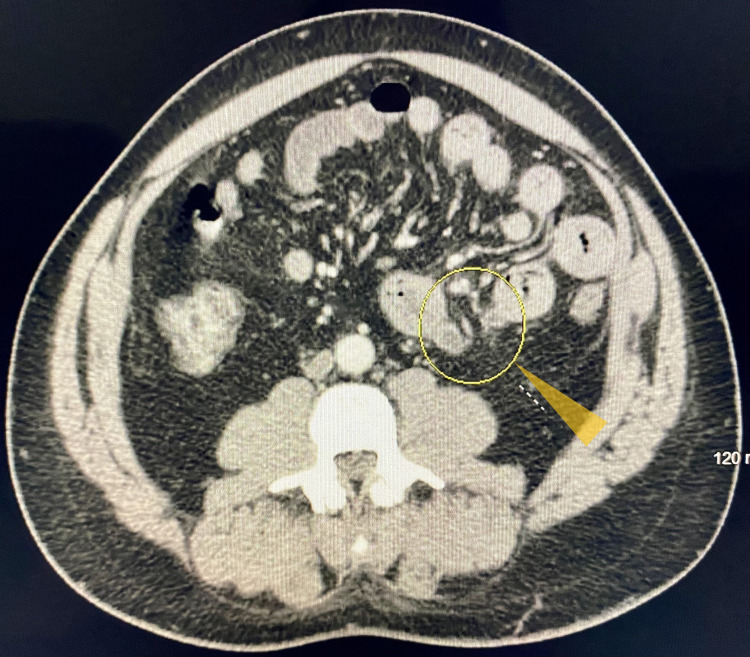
CT of the abdomen and pelvis with contrast. Showing an inflamed jejunal diverticulum in the central abdomen near the level of the aortic bifurcation and pelvic inlet (yellow triangle) consistent with jejunal diverticulitis.
